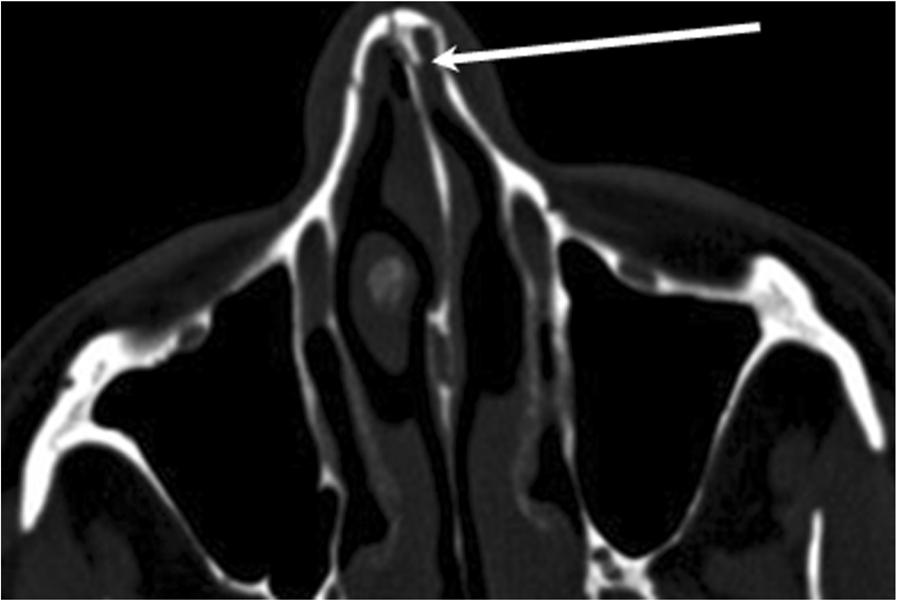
Nasal bone fracture. Axial unenhanced CT image shows nondisplaced nasal bone fractures extending to the anterior bone septum (arrow)
